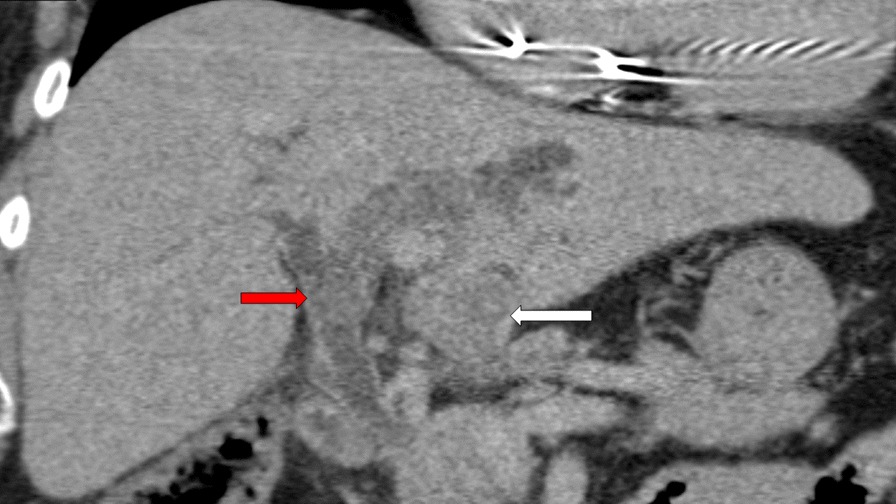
Multiphasic computed tomography scan showed a 3.1 cm lesion in segment 3 of the liver with arterial enhancement and delayed washout suspicious for HCC (white arrow), and a dilated intra- and extra-hepatic biliary tree with thickening and enhancement of the ductal wall as well as a hyperdensity in the proximal left hepatic duct (red arrow) suggestive of choledocholithiasis
